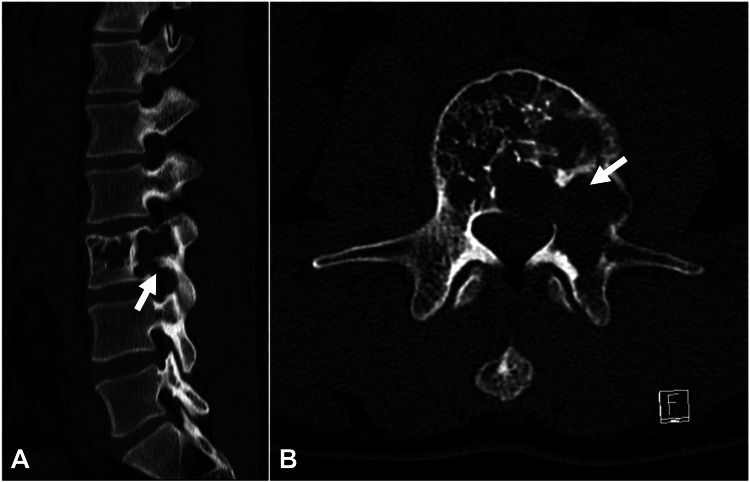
Reconstructed computed tomography. Expansile and destructive bone lesion at the vertebral body and left pedicle of the L3 (arrows). (A) Sagittal view. (B) Axial view.
